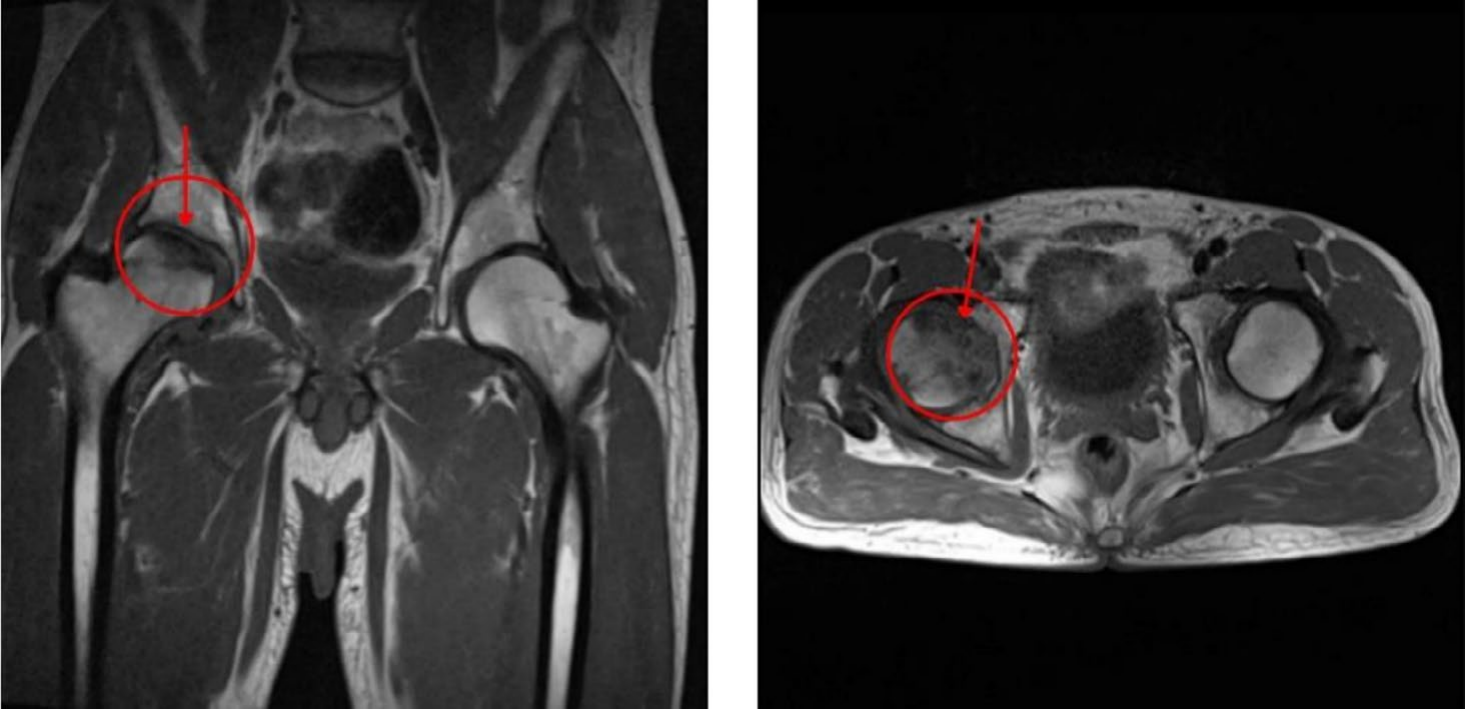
Pelvic MRI, evidence of subcortical crescent‐like signal abnormality in the right femoral head is noted due to osteonecrosis (Avascular necrosis (AVN)). Evidence of bone marrow edema at the right femoral head is seen. Mild effusion in the right hip joint is noted.
